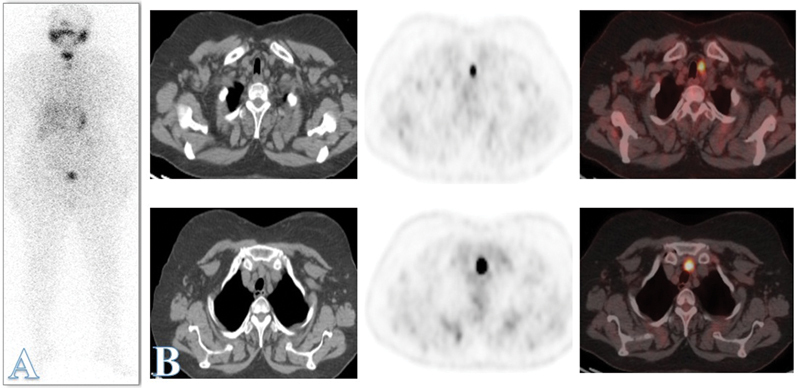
A 60-year-old female patient with papillary thyroid carcinoma underwent total thyroidectomy and received 100 mCi of RAI-131 for ablation followed by thyroxine suppressive therapy. At 6 months of follow-up, her stimulated thyroglobulin (Tg) level was 13 ng/mL with no evidence of any structural disease residue/recurrence on diagnostic RAI-131 whole-body scan or neck ultrasound (US) or computed tomography (CT) chest. (
